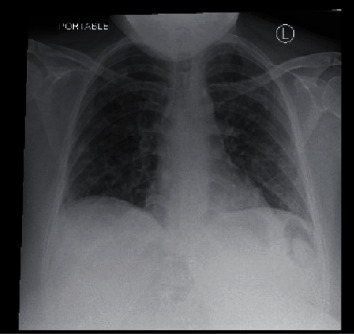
Chest X-ray on arrival showing diffuse bilateral opacities.
